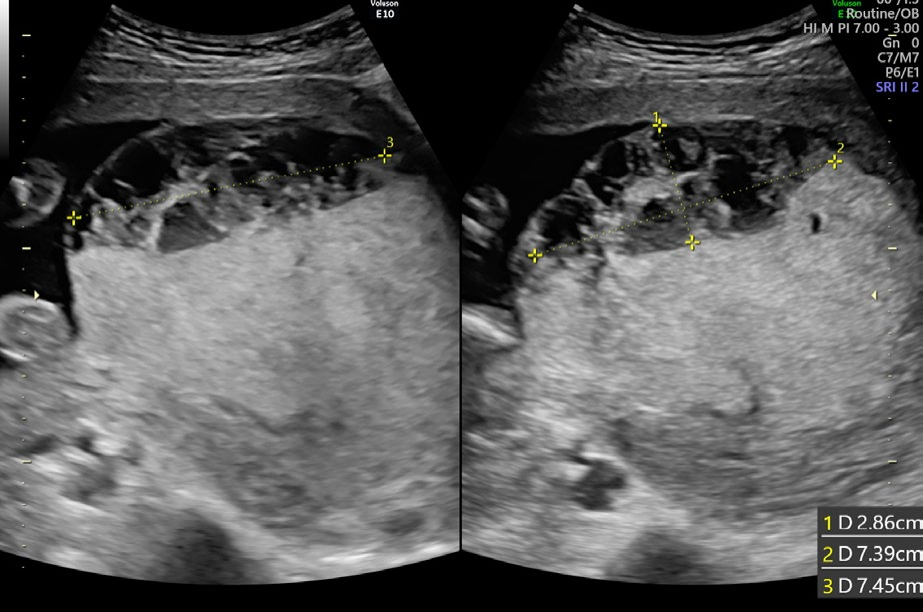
Ultrasonographic image shows intraplacental fluid‐fluid level (24 wk 4 d of gestation). Color Doppler sonography showed no blood flow in the reticulate sonolucent areas
